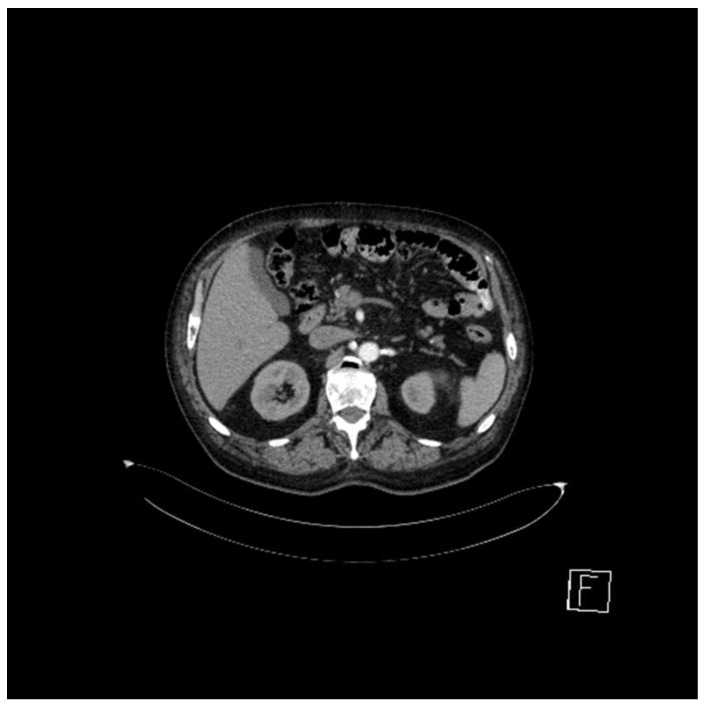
Computed tomography (January 2022): hypocapturing hepatic lesions, two of them with a stationary appearance compared to September 2021 and one not visualized upon the previous examination. For additional characterization. an abdominal MRI with intravenous contrast medium was recommended. No new secondary brain or pulmonary determinations were observed. Secondary osteocondensed bone determinations, some of them with slight dimensional progression, were noted.
